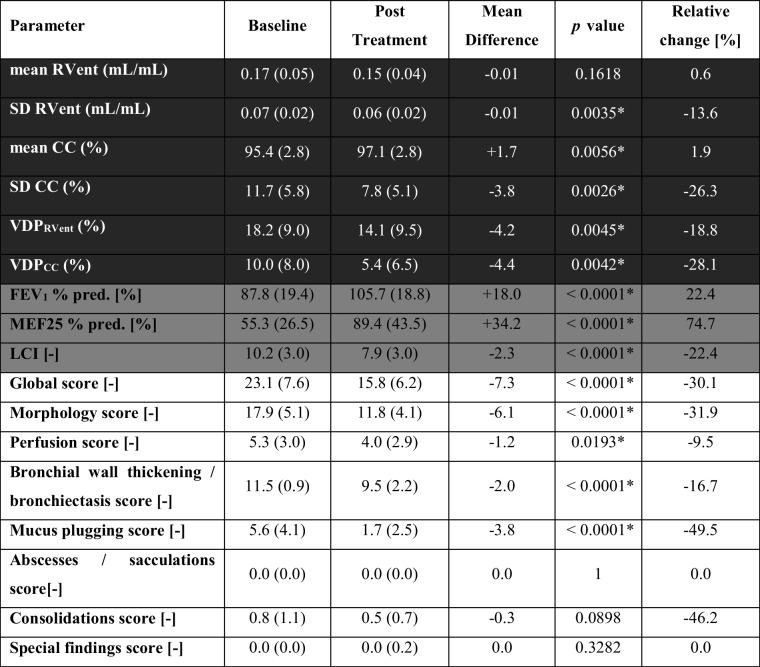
Analysis of 3D PREFUL, spirometry, N_2_-MBW, and morphological MRI parameters in response to ETI therapy. Baseline and post-treatment ventilation values are expressed as average with standard deviation in brackets. Significant results are marked with *
